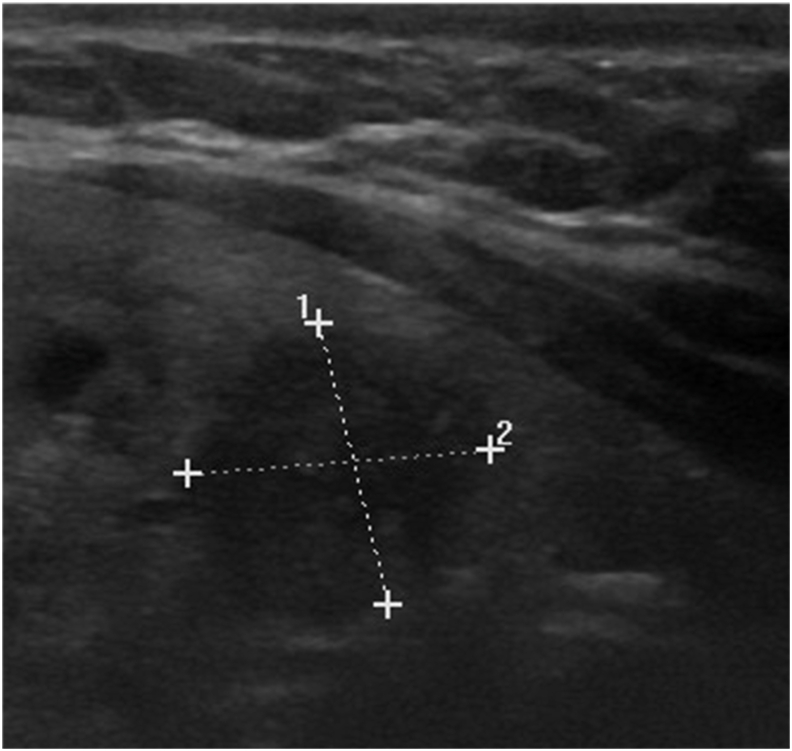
Ultrasounds showed a hypoechoic and plunging nodule tirads 5, 35*25MM.
